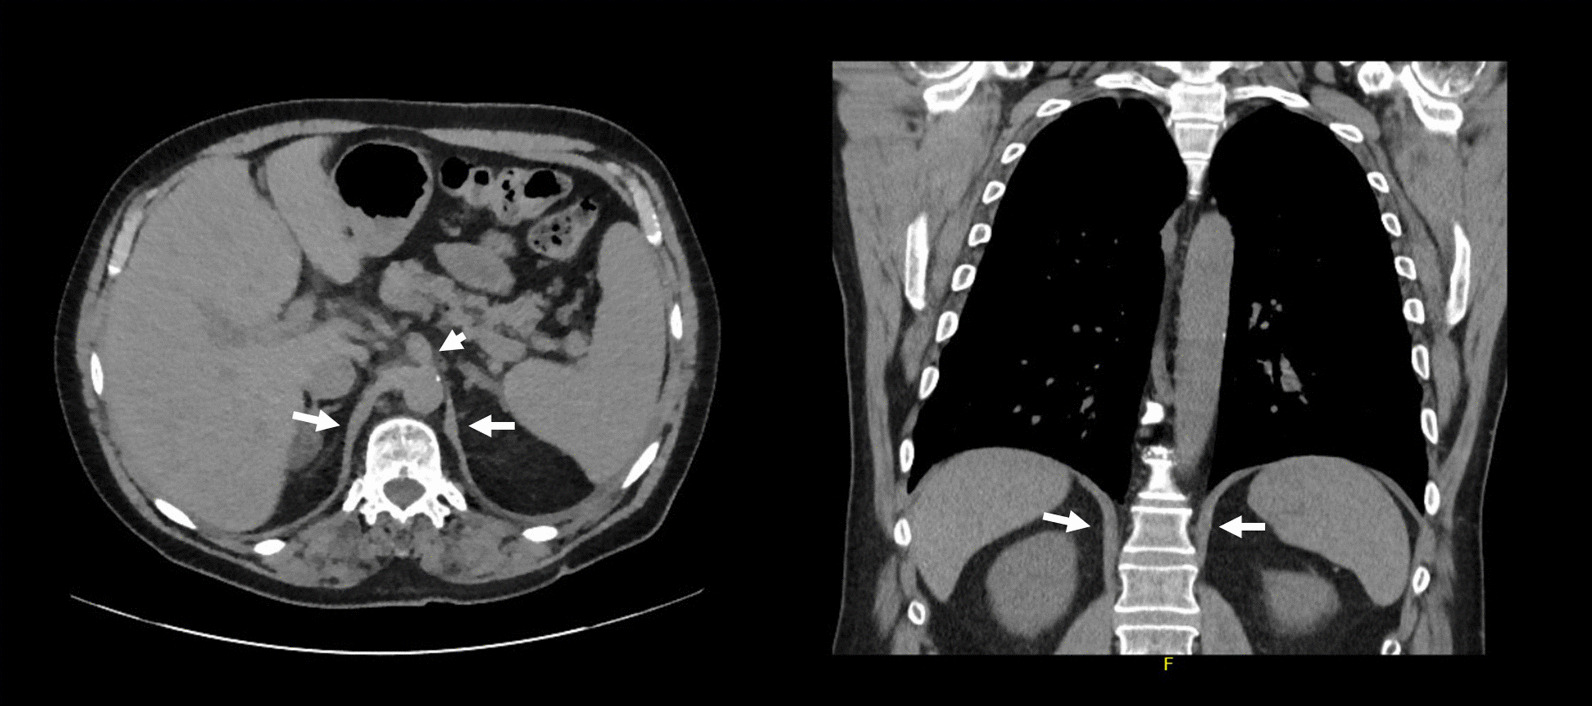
Assessment of crural diaphragm thickness by chest CT using axial and coronal images. (*Left panel*) With axial imaging, the thickness of the right and left crural hemidiaphragms (arrows) can be measured at level of the origin of the celiac artery (arrowhead). Note the nodularity of the left crus, a normal variant of the diaphragm’s shape. (*Right panel*) With coronal imaging, the thickness of the crural hemidiaphragms (arrows) can be measured at level of the first lumbar vertebra
